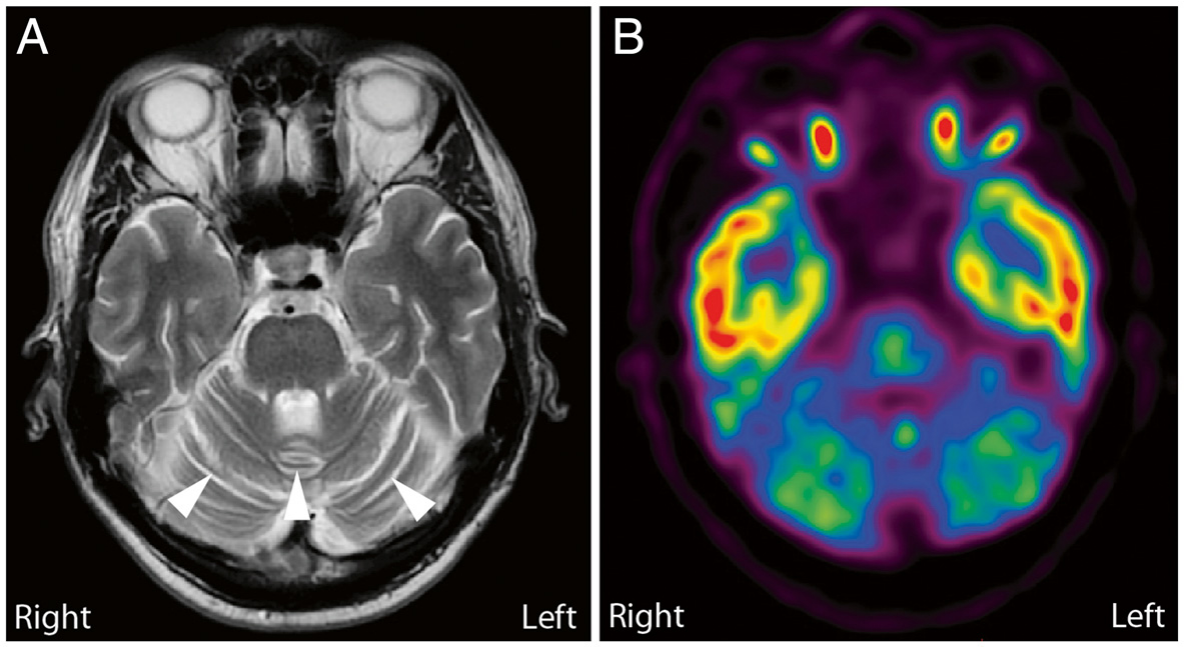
Brain MRI and^18^ F-FDG-PET-scan of the proband in the transverse plane. A: The MRI scan included a diffusion weighted sequence (DWI) and a T2* weighted sequence. No focal lesions were observed; however, the cerebellar hemispheres and vermis appeared atrophic (arrow heads). B: ^18^ F-FDG-PET-scan showing strikingly reduced metabolism in the cerebellum suggesting pathology primarily localized to the cerebellum.
